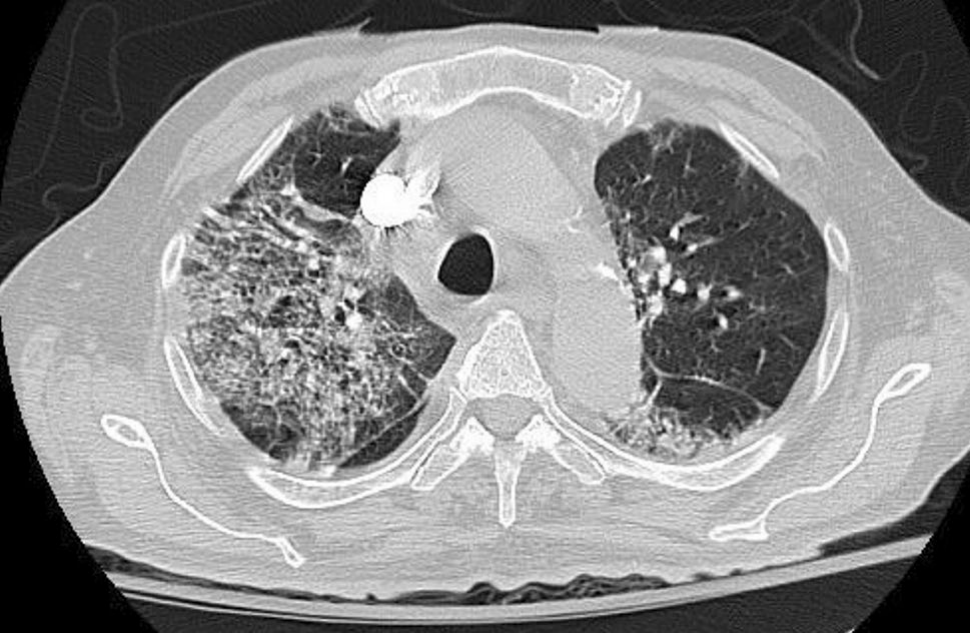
CT of chest upon admission
